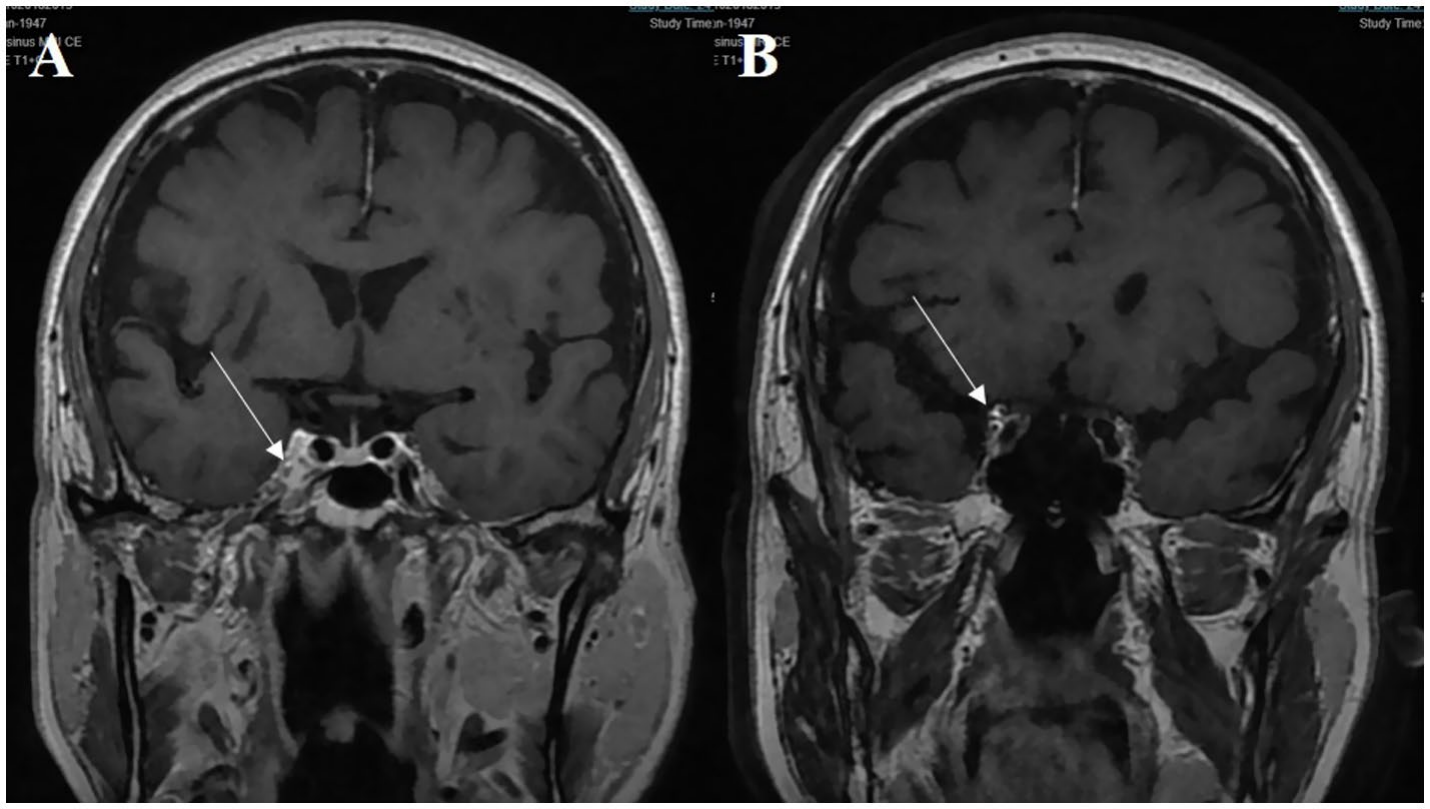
A 70-year-old male patient presenting with third cranial nerve palsy. (A) The abnormal asymmetric high intensity was found in the cavernous sinus on the post-gadolinium coronal T1-weighted image (white arrow). (B) The lesion extends toward the ipsilateral orbital apex on the coronal enhanced T1-weighted image (white arrow).
